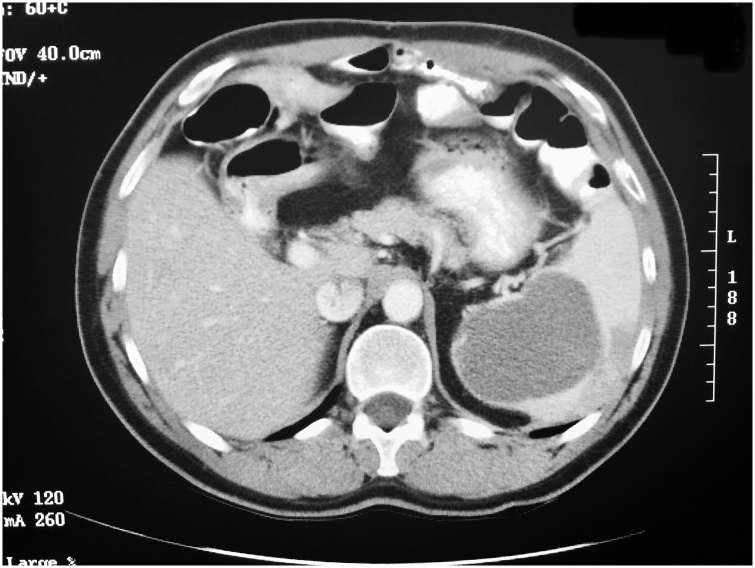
A 48-year-old man developed right lower quadrant pain and was taken to the operating theater. A ruptured appendix was associated with widespread mucinous tumor within the peritoneal space. He was treated with 5-fluorouracil plus leucovorin chemotherapy. Seven years after the appendectomy a repeat CT scan shows a 7 cm in diameter cystic mass within the central portion of the spleen.▪On November 24, 2004, exploratory laparotomy was performed. The mucinous adenocarcinoma was contained within the splenic capsule. The spleen with a portion of the left hemidiaphragm was removed intact. No HIPEC or EPIC was used.▪On March 8, 2013, the patient died but cause of death was unknown.
